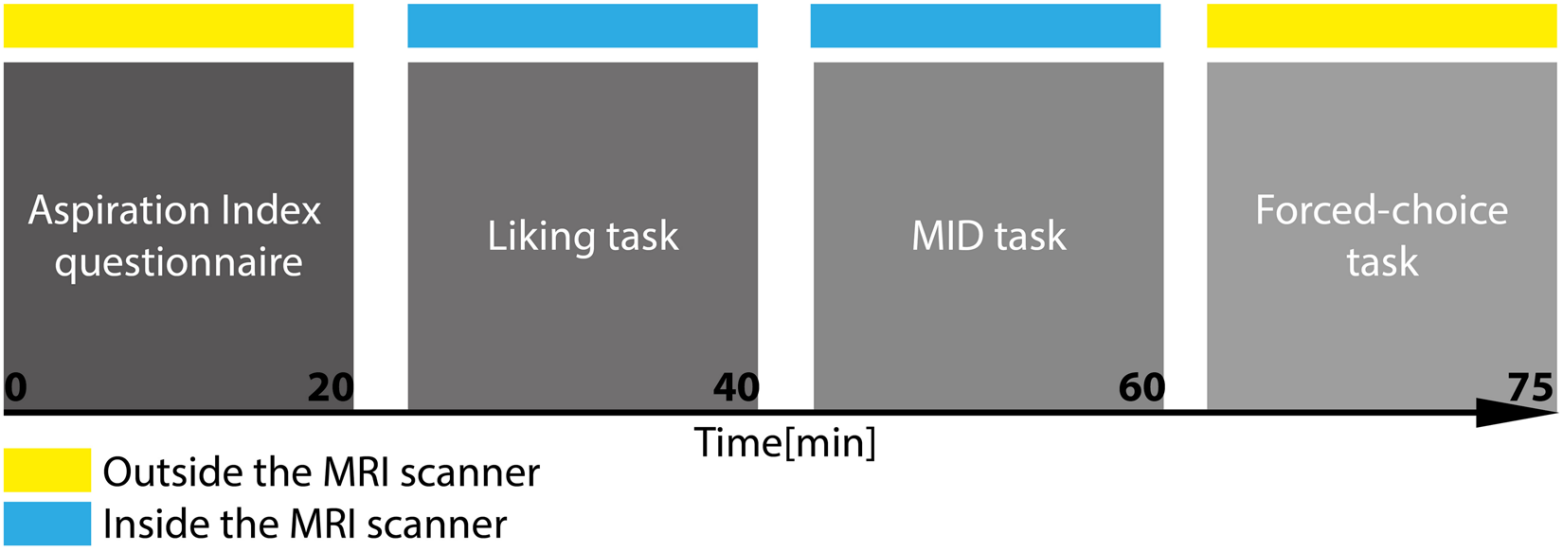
Experimental timeline for a complete session in minutes (X axis) for one participant, showing the order of task presentation and specifying whether the task was performed inside (blue rectangles) or outside the MRI scanner (yellow rectangles). MID: Monetary Incentive Delay task.
